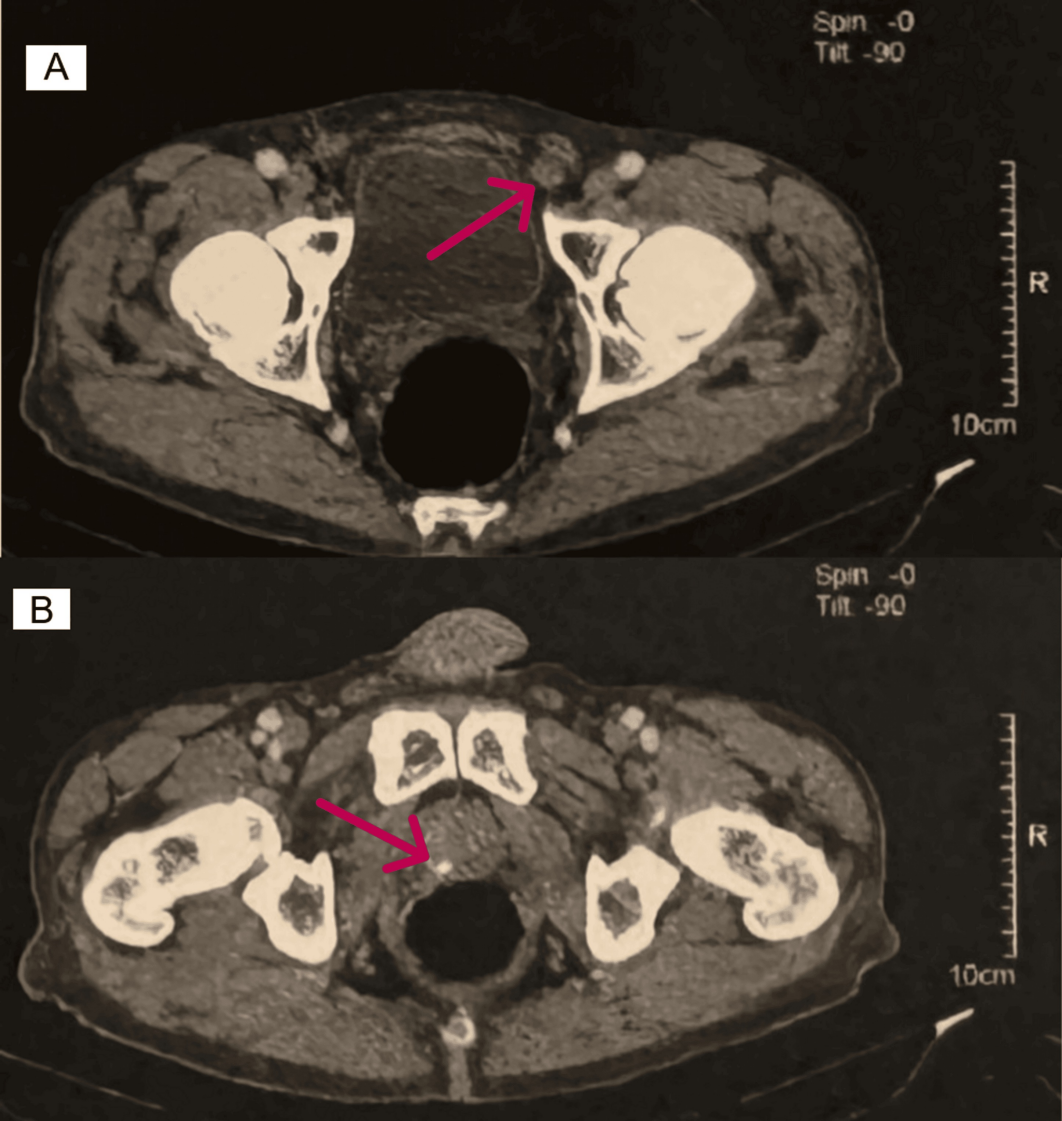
Pelvic CT showing right iliac lymphadenopathy and prostatic lesion (A) Axial CT showing a 29 mm right iliac lymphadenopathy (red arrow). (B) Axial CT demonstrating a 13 mm enhancing lesion (red arrow) in the right peripheral zone of a normal-sized prostate, consistent with the primary tumor. CT: computed tomography
